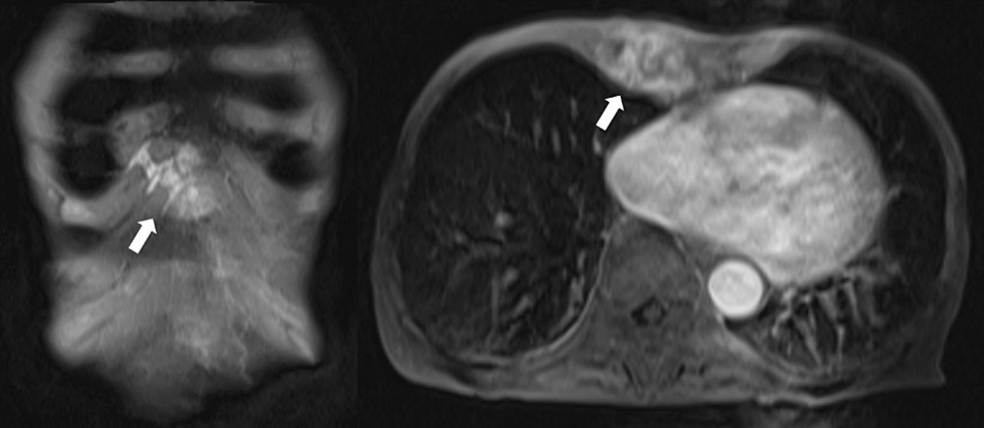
Chest MRI findings. MRI showed melting images of the right fifth, sixth, and seventh costal cartilages and surrounding mass formation (white arrow).
